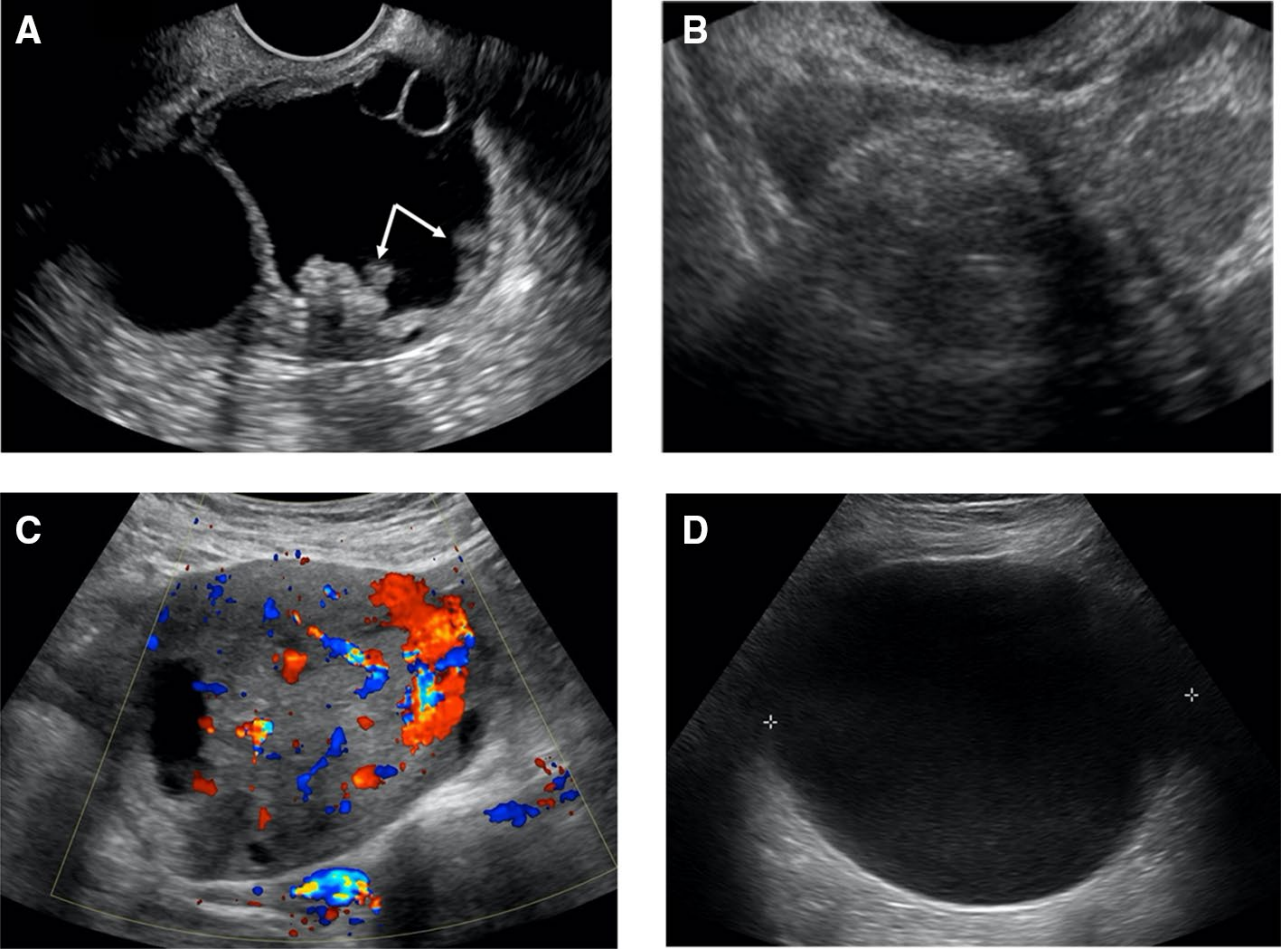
Ultrasound Examples of IOTA Features. A Grayscale image of an ovarian multilocular cystic lesion with multiple (≥ 4) papillary projections (white arrows). This is an example of M3: ≥ 4 papillary projections, which had almost perfect interobserver agreement. B Grayscale image of an ovarian echogenic lesion with acoustic shadows (example of B3), which had almost perfect interobserver agreement. C Color Doppler image of an ovarian solid lesion with very strong blood flow (example of M5), which had substantial interobserver agreement. D Grayscale image of an ovarian unilocular cyst (example of B1), which had moderate interobserver agreement
